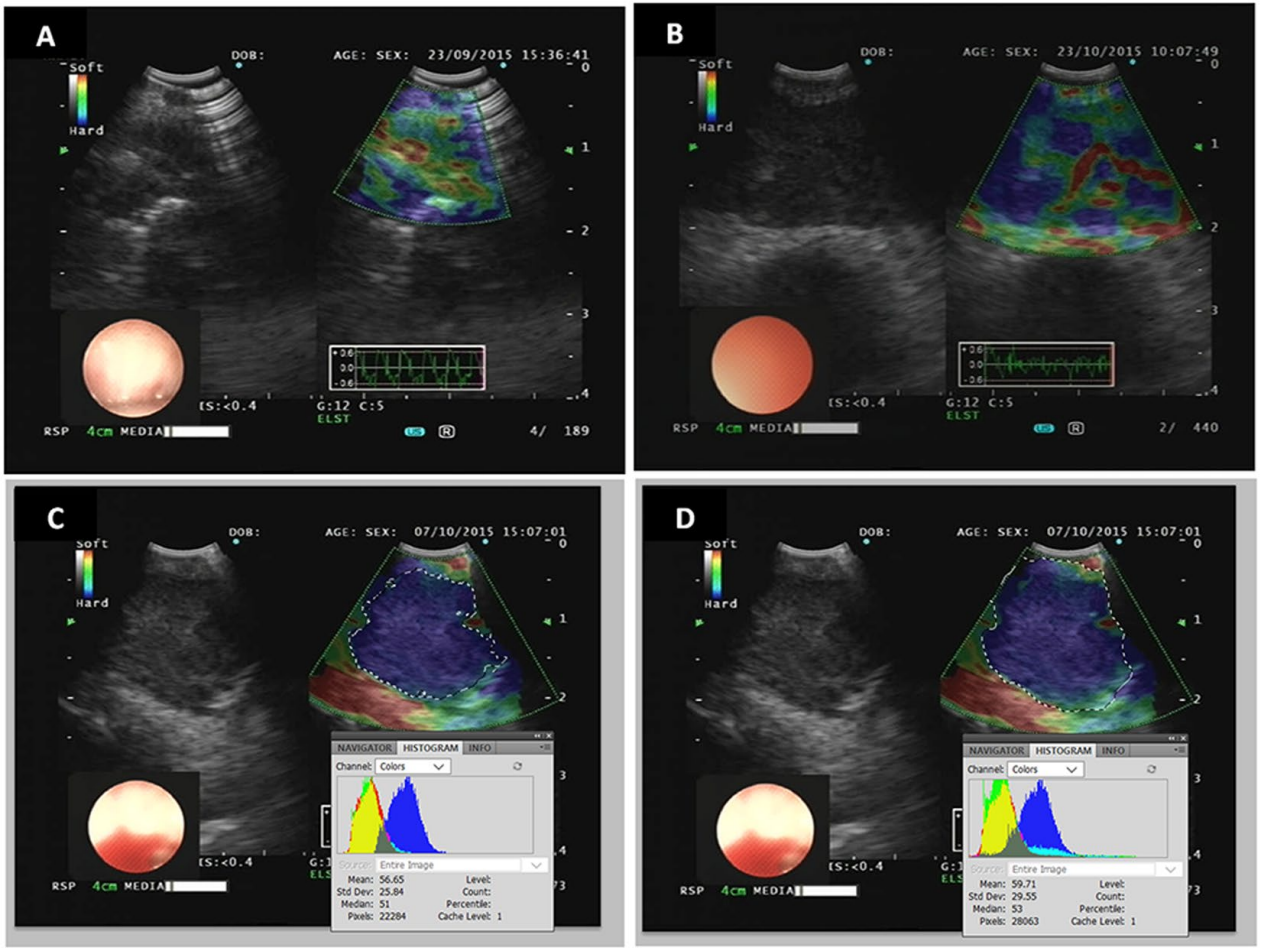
The representative color patterns of LNs on EBUS elastography imagings. For every elastogrpahy imaging like picture A, the LN was shown both by B mode ultrasound on the left and corresponding elastography imaging on the right. In the elastography image, the blue, green and red color indicated the hardest, medium and softest tissues respectively. (A) LN with BCP <36.7%. (B) LN with BCP > 36.7%. (C) Photoshop showed the pixels of blue color in another LN, (D) (same patient as C): Photoshop showed the pixels of the whole LN area. BCP was calculated by dividing the pixels of blue color in picture C with that in picture D. EBUS, endobronchial ultrasound; LN, lymph node.
